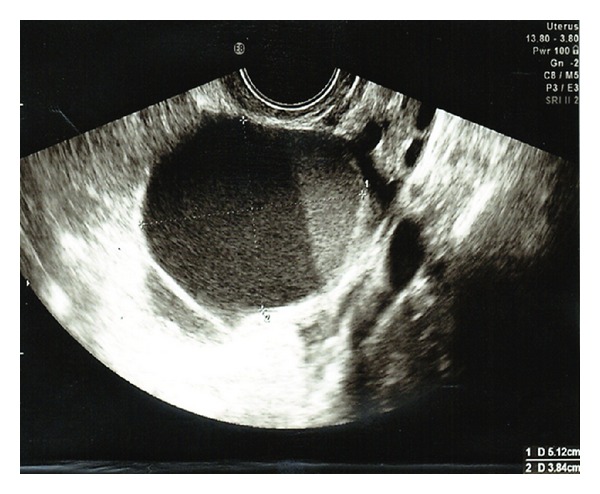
Pelvic ultrasound, showing a large complex ovarian cyst with layered low level echoes measuring 5.1 × 3.8 cm.
